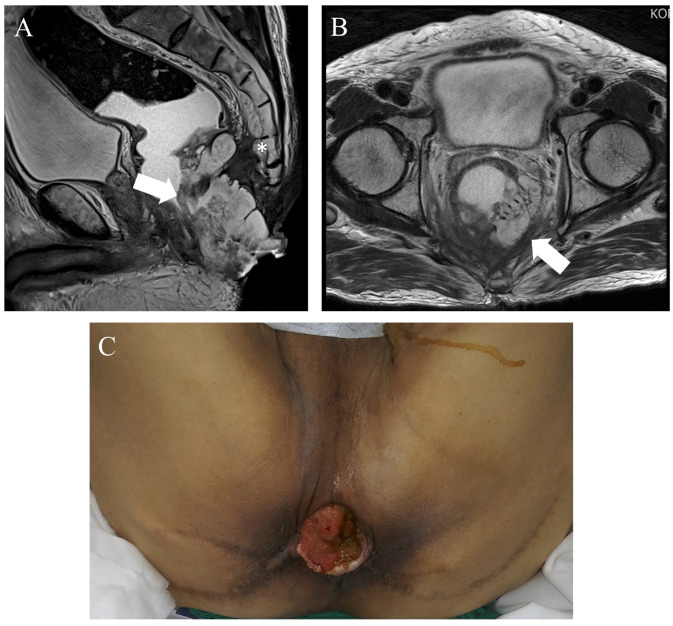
(A,B) CT scans and intraoperative view (C) of an extensive anal cancer (arrows) with infiltration of the presacral area (white star).
